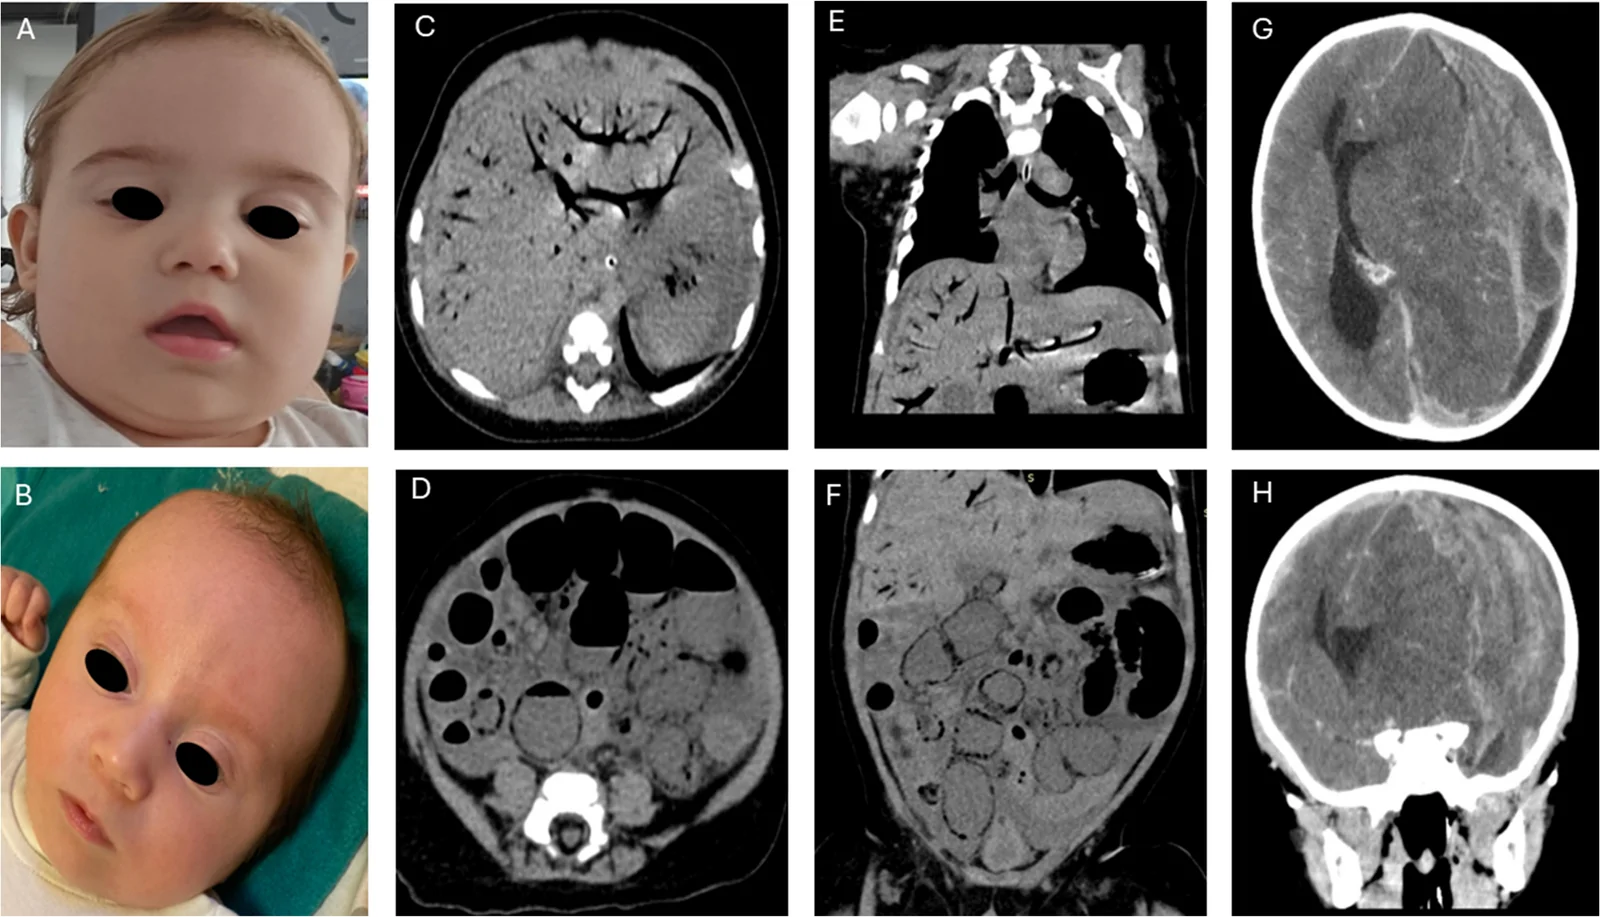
Clinical and radiologic features in patients with TRNT1 deficiency. (A–B) Facial dysmorphism observed in the two patients, demonstrating frontal bossing, midface hypoplasia, and downslanting palpebral fissures. (C–F) Abdominal CT imaging demonstrating portal venous gas, pneumatosis intestinalis, and dilated bowel loops consistent with severe bowel ischemia. (G–H) Cranial CT demonstrating extensive subdural hematoma with significant mass effect and associated subarachnoid hemorrhage
